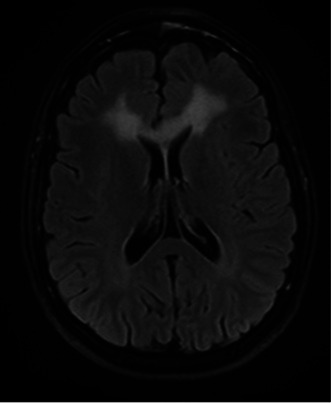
FIGURE 1 Brain MRI (axial FLAIR) obtained at age 34 showing diffuse white matter hyperintensity involving both cerebral hemispheres, mainly in periventricular regions, corpus callosum, and posterior hippocampal areas.
